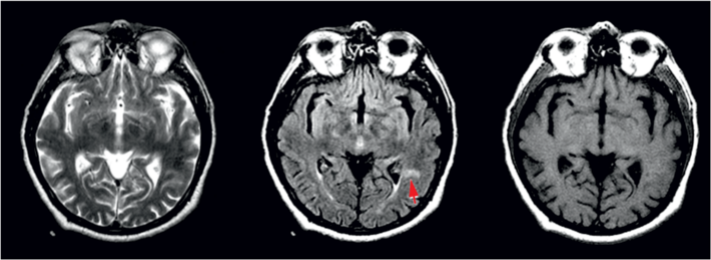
Paraventricular left temporal poligonal area with reduced dimensions between exams - area of ischemia in the subacute stage - hyperintense on T2 and FLAIR, hypointense on T1 without restricted diffusion
